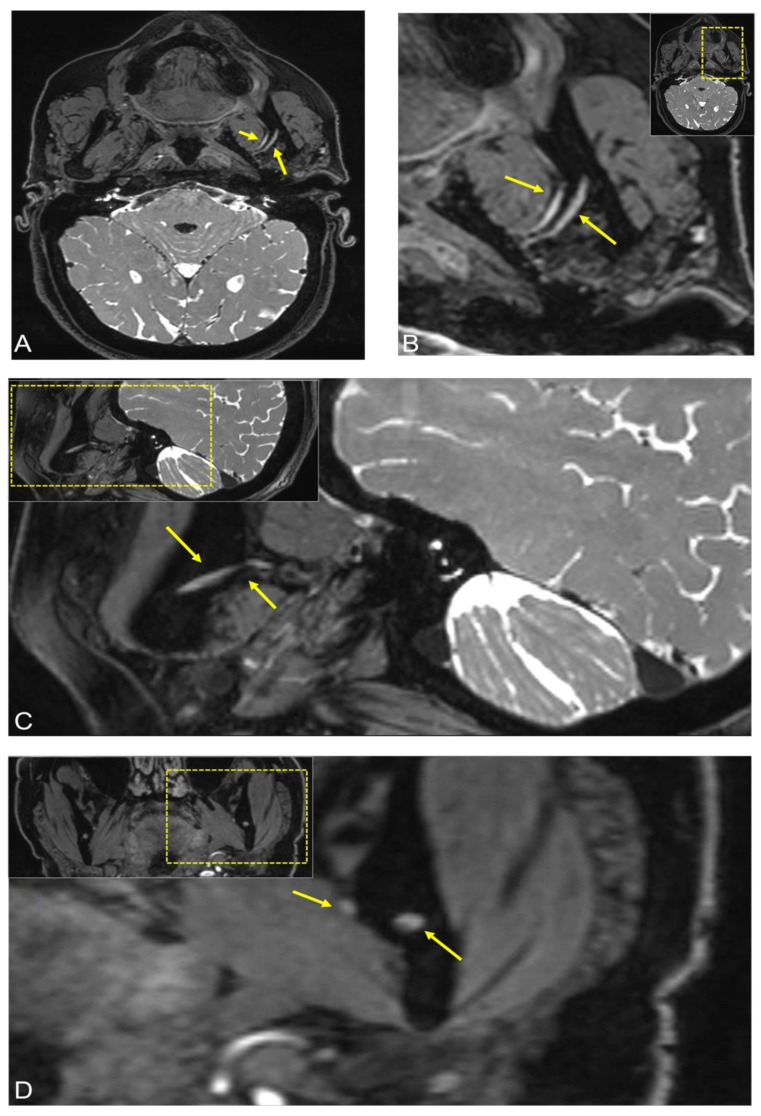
Follow-up MRI after three months of the same patient. The 3D Double-Echo Steady State (DESS) MRI protocol revealed the following: (A,B) Axial, (C) sagittal, and (D) coronal side-by-side comparison of the image reconstructions showed bilateral symmetry of the mandibular nerve with a loss of signal intensity in the 3D-DESS sequence compared with the previous examination, no longer indicating evident pathological signal alterations. In all images, the short arrow shows signal hyperintensities of the lingual nerve at the anatomic point where it begins to pass from lateral to medial and the longer arrow represents the inferior alveolar nerve before it enters into the mandibular foramen. For orientation, the dotted rectangles in the corner show the enlarged area.
